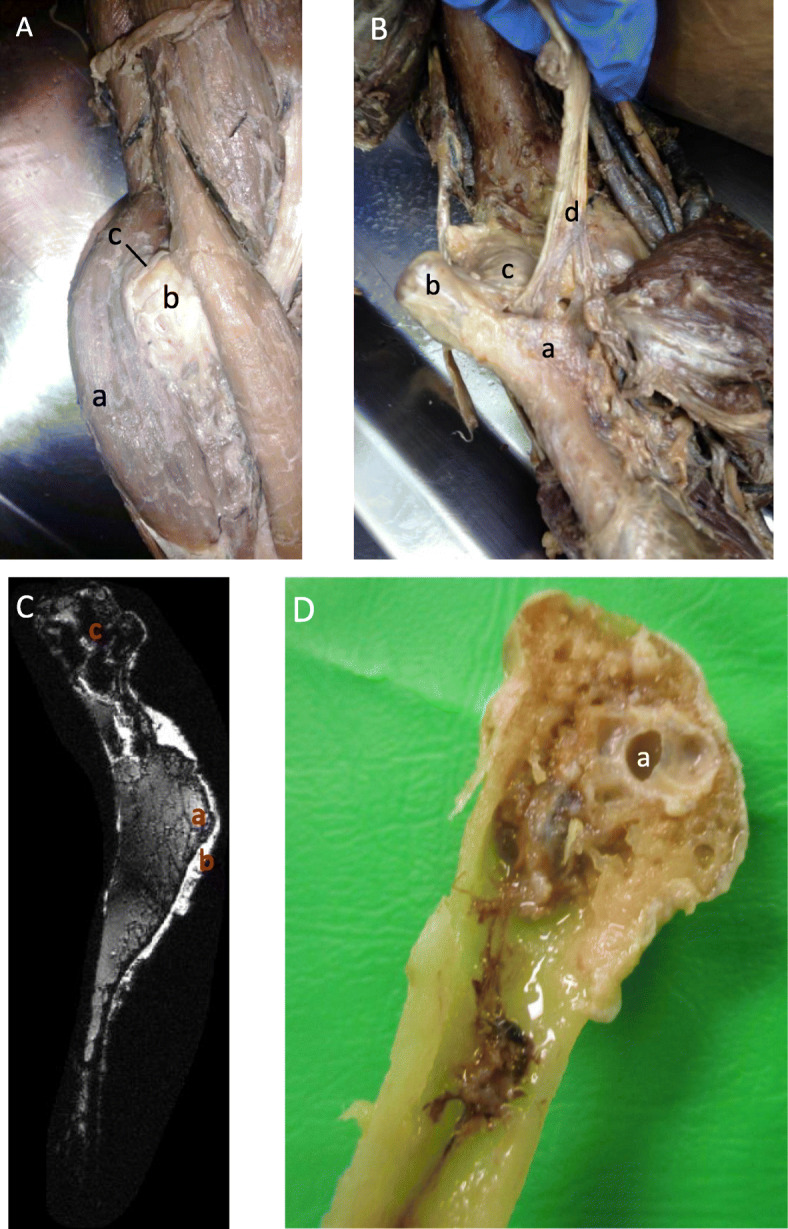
Right proximal forearm. a Superficial dissection. A tumour has caused lateral displacement of the brachioradialis muscle (a). The radial nerve (c) has been diverted over the superior surface of radial head (b). b Deep dissection. Exostosis in the region of the radial tuberosity (a), radial head dislocation (b). The capitulum (c) formed an articulation with the radius at the radial tuberosity (a), causing the biceps brachii tendon (d) to be incorporated into the joint. c DESS MRI of proximal radius. An osteochondroma present at the radial tuberosity (a) has a continuous cortex and medulla with the underlying bone. A cartilage cap is present on the surface (b). Note the low signal within the head of the radius (c). d Sagittal section of radial head. Radius displays a large void (a) within the epiphysis
